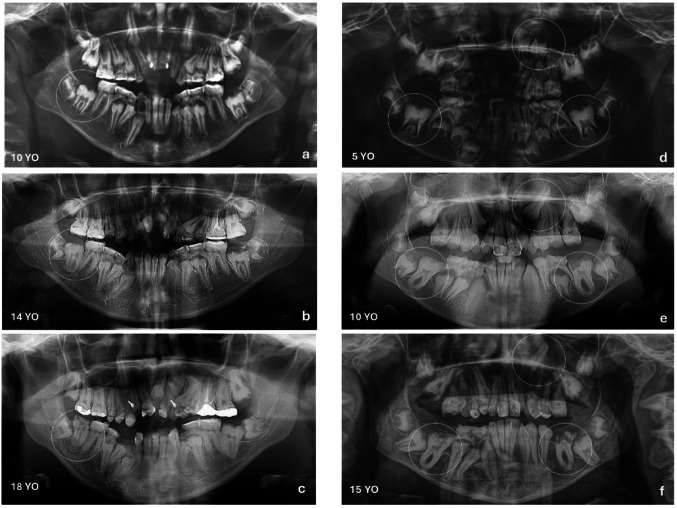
Longitudinal panoramic radiographic evaluation of Patient 1 a–c and Patient 2 d–f. a Panoramic radiograph of Patient 1 at age 10 shows unerupted teeth 47 (circled) without detectable resorptive changes and preserved crown morphology. a At age 14, PECR can be noted due to the irregular coronary surface, and at age 18, tooth 47 had already erupted, but note the extensive coronary destruction not compatible with caries decay c (see also the clinical aspect in Fig. 1d). d Panoramic radiograph of Patient 2 at age 5 reveals normal teeth development. Teeth presented normal crown morphology and surface. Teeth 23, 36, and 46 are circled. e At age 10, observe the unerupted teeth 23, 36, and 46 with initial signs of PECR. f At age 15, the progression of PECR is clearly observed
